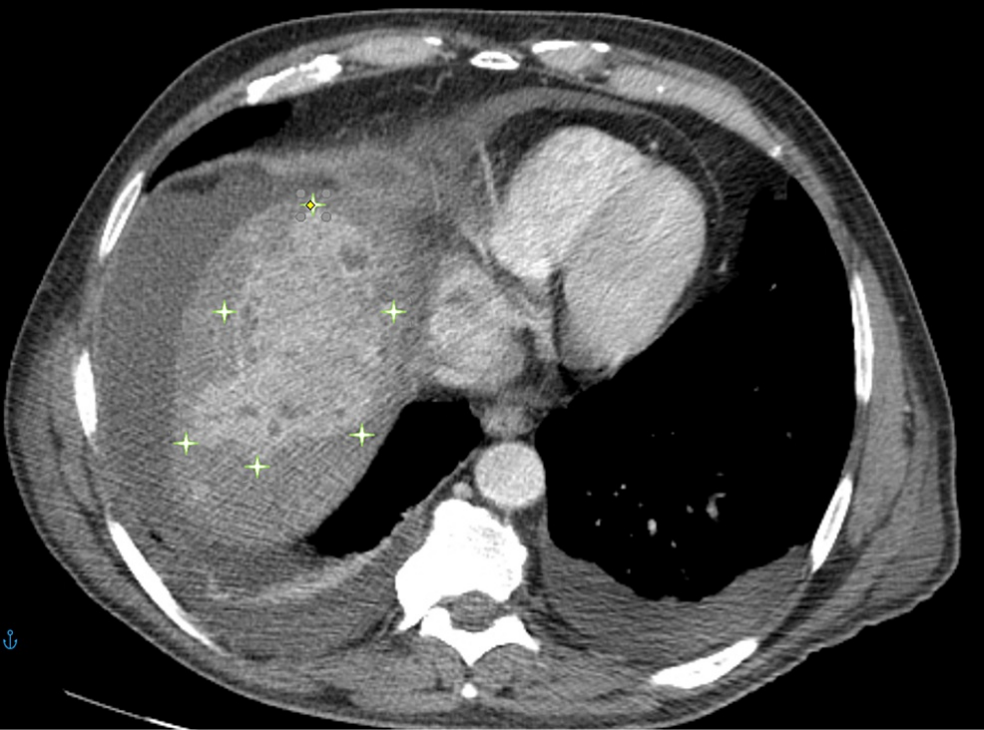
Computerized tomography (CT) with angiography Computerized tomography with angiography showing a liver mass compatible with hepatocarcinoma (outlined by the stars).
